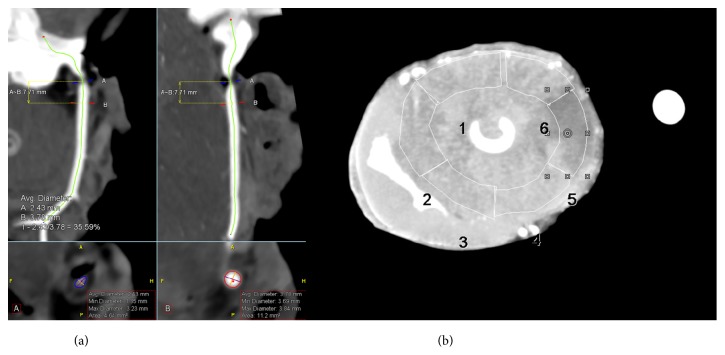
Example of images of the Cx artery with an FFR of 0.30. (a) The computed tomography angiography (CTA) image in curved multiplanar reformat shows the luminal narrowing. (b) The resulting perfusion defect in segment 5 as visible on maximum intensity projection (MIP) image.
